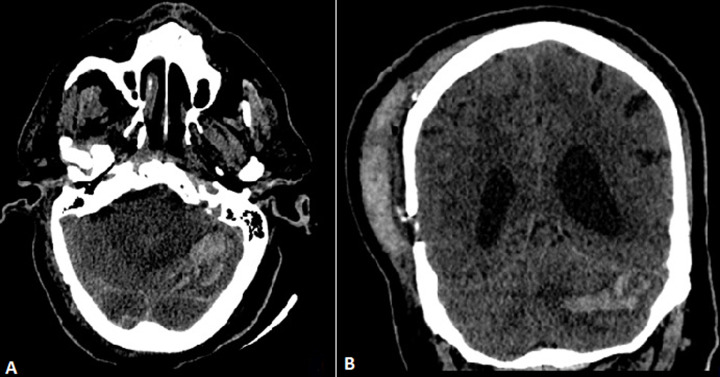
axial and coronal non-contrast CT scan of the brain on the second postoperative day revealed a cerebellar hemorrhage, dominating in the right cerebellar hemisphere and the vermis
